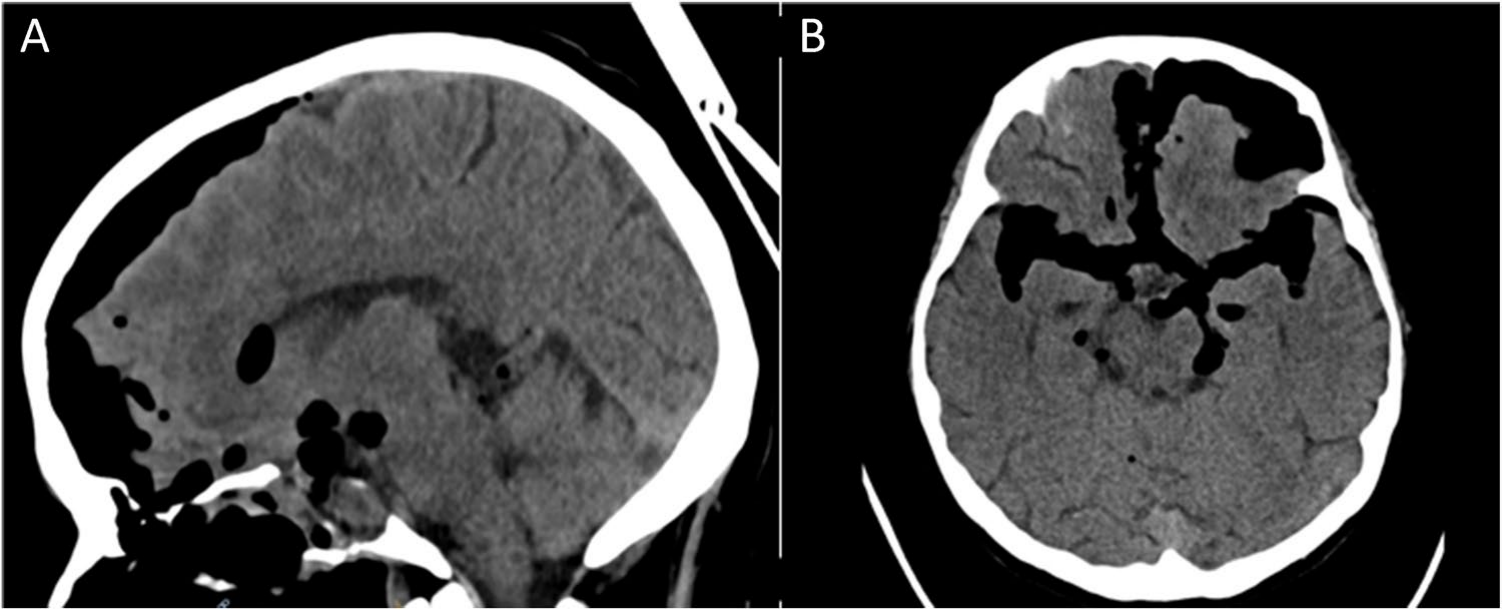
Preoperative CT patient 2 showing pneumocephalus due to CSF leakage after previous surgery in A sagittal view and B axial view
